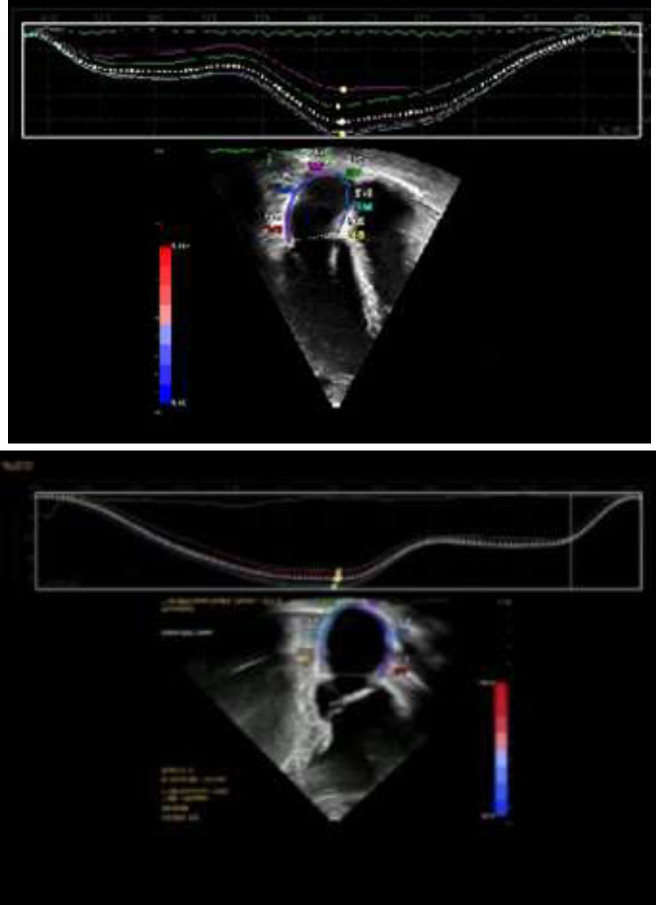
LA strain curve: Two‐dimensional speckle tracking strain demonstrating phasic LA strain (LASr, LAScd, and LASct) from the apical 4 chamber view and apical 2 chamber view. Segmental strain from 6 segments as well as LA global strain (white dotted line) is shown
